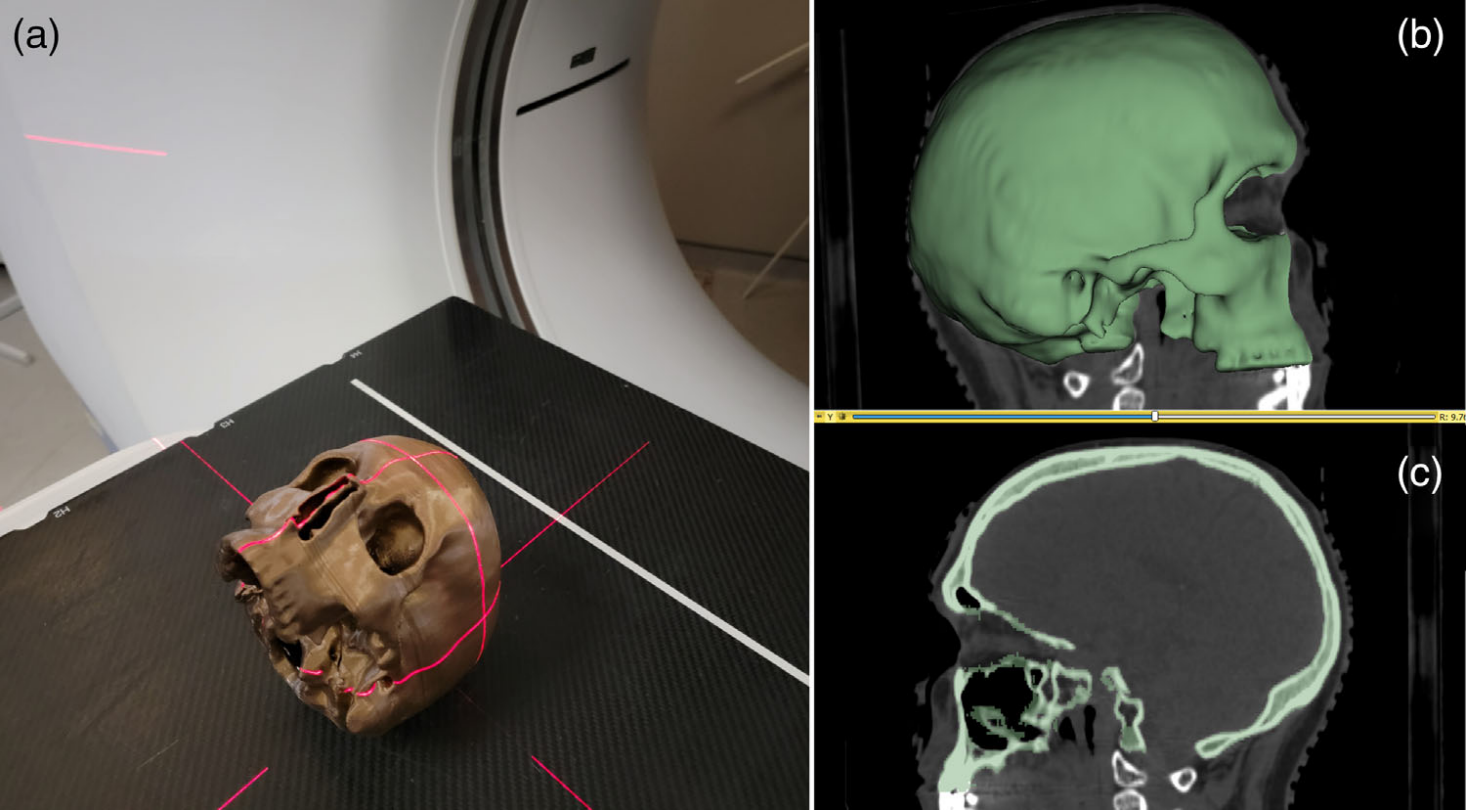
(a) The printed skull aligned on the computed tomography (CT)‐bed for imaging. (b) The 3D model of the skull embedded in a sagittal view of the skull. (c) A sagittal view of the skull with the contoured regions highlighted in green
